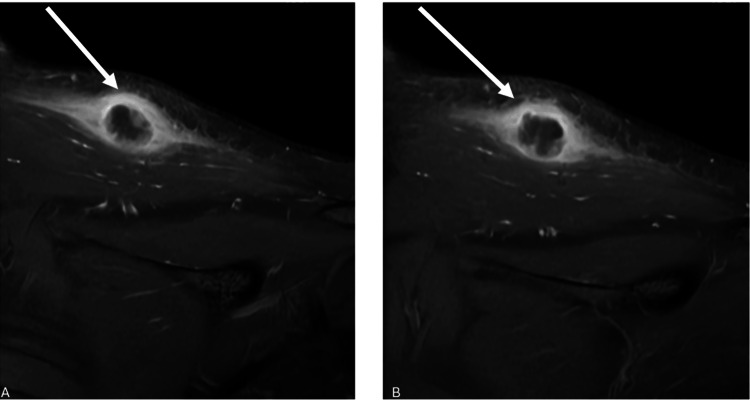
(A-B) Coronal magnetic resonance images of the patient. The white arrow shows the lesion in the relevant magnetic resonance images.
